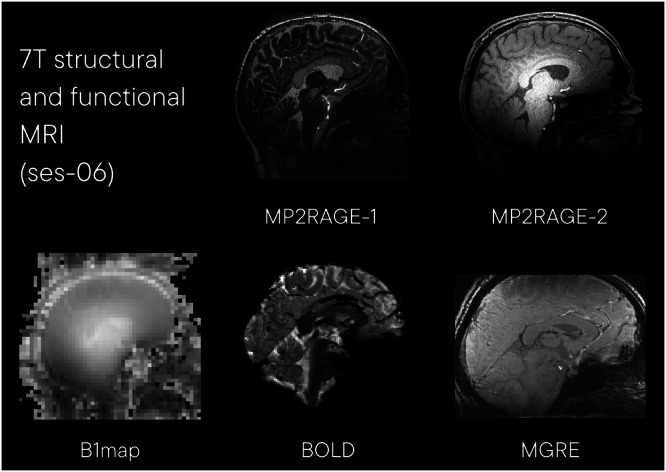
Example images from session 06, 3 T functional and perfusion MRI. MP2RAGE: magnetization prepared 2 rapid gradient echoes; B1map: B1 inhomogeneity map; BOLD: blood oxygen level dependent functional MRI; MGRE: Multi-echo 3D gradient echo.
